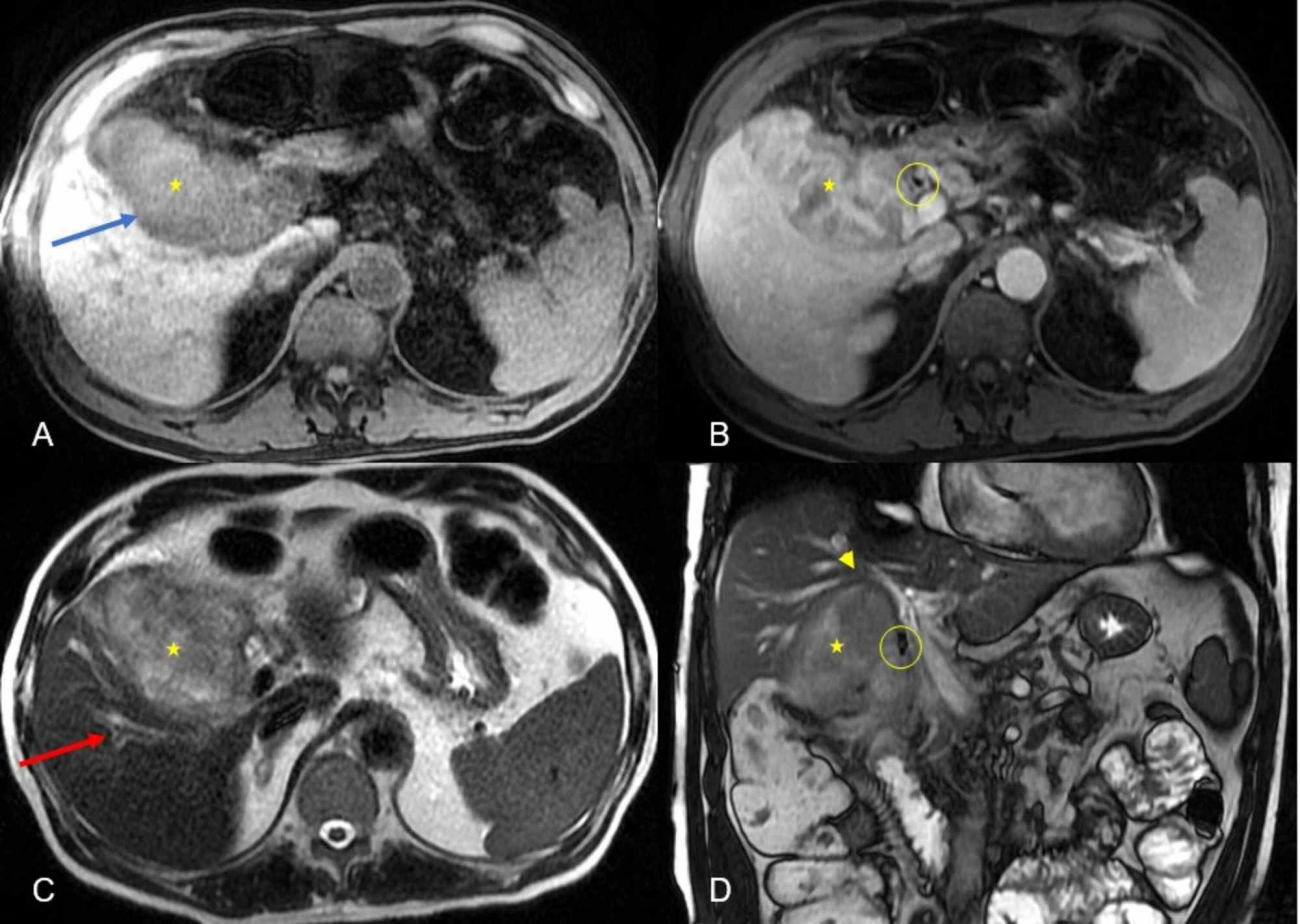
Infiltrative pattern and involvement of porta hepatis. Multiple abdominal MRI images: (A) axial T1 precontrast with fat suppression; (B) axial T1 postcontrast with fat suppression; (C) axial T2 and (D) coronal 2D FIESTA demonstrating a large lobulated mass in the gallbladder fossa (yellow star). On axial T1 image (A), the mass appears hypointense while on axial T2 image (C), it appears heterogeneously hypo/hyperintense (yellow star). The mass shows heterogeneous enhancement on postcontrast T1 image (B). There is infiltration of the inferior aspect of the right hepatic lobe (blue arrow on image A). The mass compresses the CBD with mild upstream right intrahepatic biliary dilation (red arrow on image C). There is compression and narrowing of the anterior branch of the right portal vein (yellow arrowhead on image D). Surgical clip (yellow circle on image D) from prior cholecystectomy. FIESTA, fast imaging employing steady-state acquisition; CBD, common bile duct
